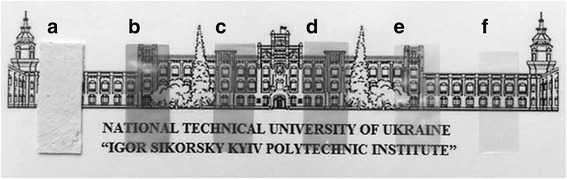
a Comparison of transparent strips made from initial OSP. b OSP after ultrasound treatment. CNF films prepared after hydrolysis by sulfuric acid of concentration 43% at (c) 20 °C, 30 min; d 20 °C, 60 min; e 60 °C, 30 min; f and 60 °C, 60 min. The duration of ultrasound treatment all patterns was 30 min
